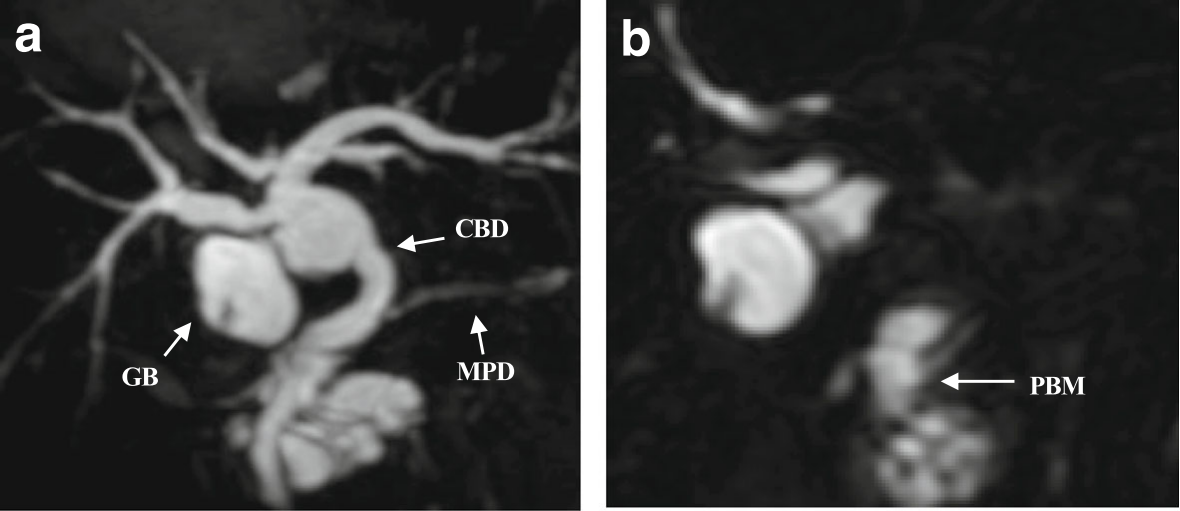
a, b Magnetic resonance imaging (MRI) showed a pancreaticobiliary maljunction with cystic dilatation of the common bile duct (type Ia [Todani classification]). GB gallbladder, CBD common bile duct, MPD main pancreatic duct, PBM pancreaticobiliary maljunction
